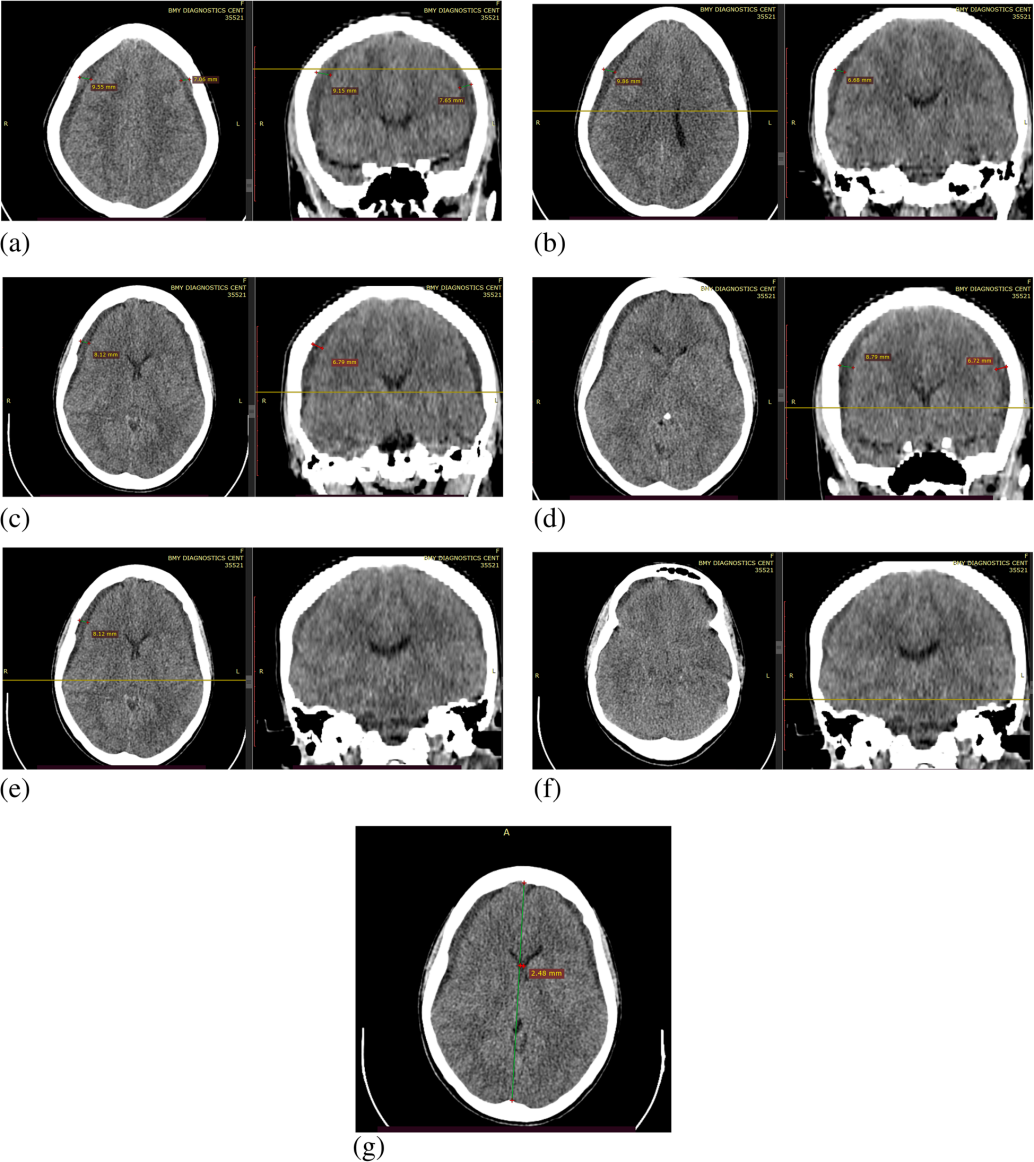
(image of patient CT scan of the patient at different levels of section as labeled from ‘a to g’)
